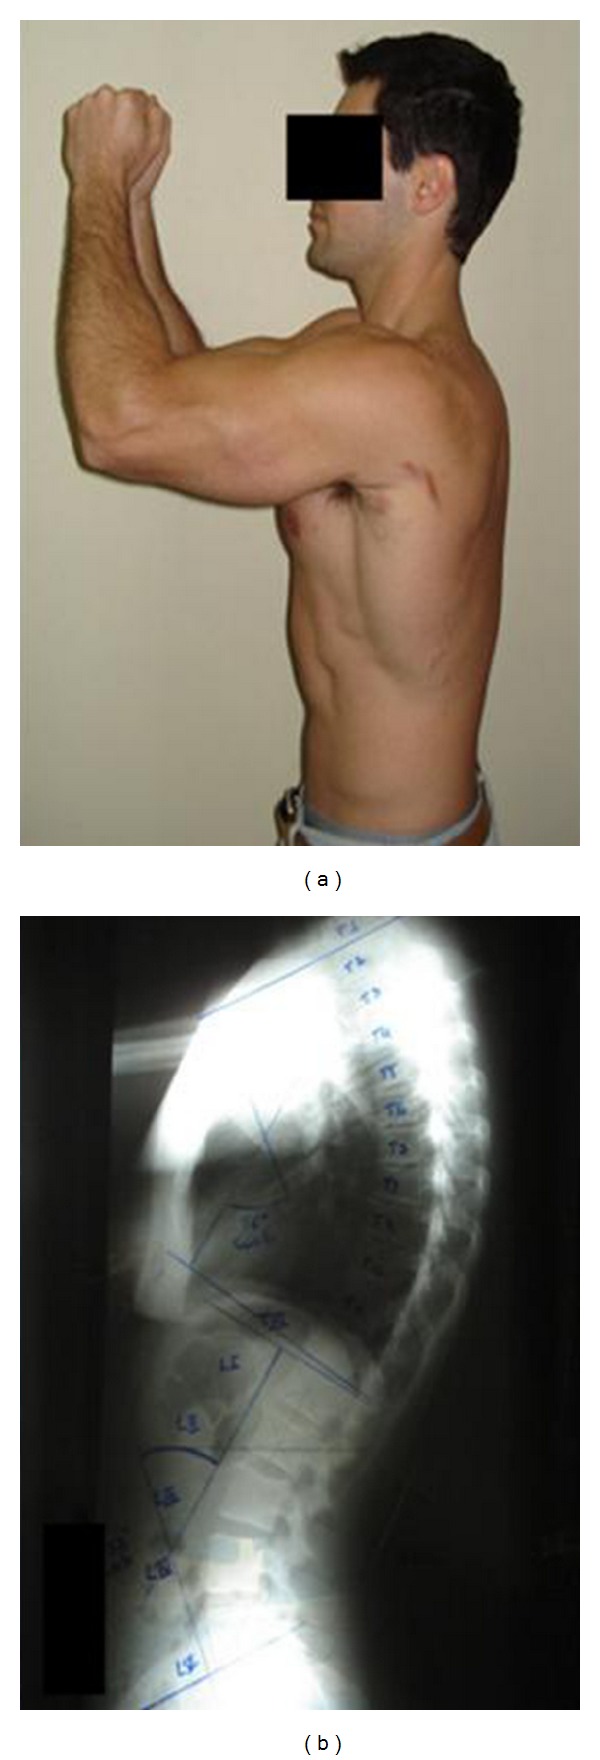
(a) Position of the subjects during both evaluation procedures; (b) X-ray image of the spine.
